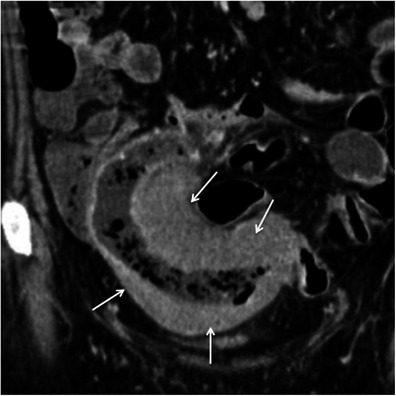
Small bowel lymphoma. Reformatted coronal contrast-enhanced CT scan shows concentric and regular segmental thickening of a small bowel loop (*arrows*) with homogeneous contrast enhancement. *Adapted from the electronic poster “Bowel wall thickening—a complex subject made simple” DOI:*10.5444/esgar2011/EE-063
